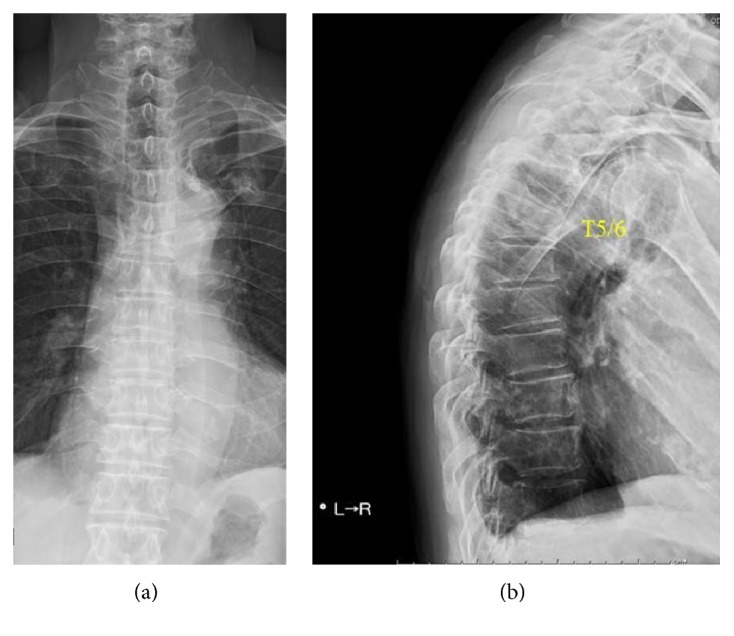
(a) Anteroposterior and (b) lateral radiographs of the thoracic spine show compression fractures of the T5 and T6 vertebrae.
